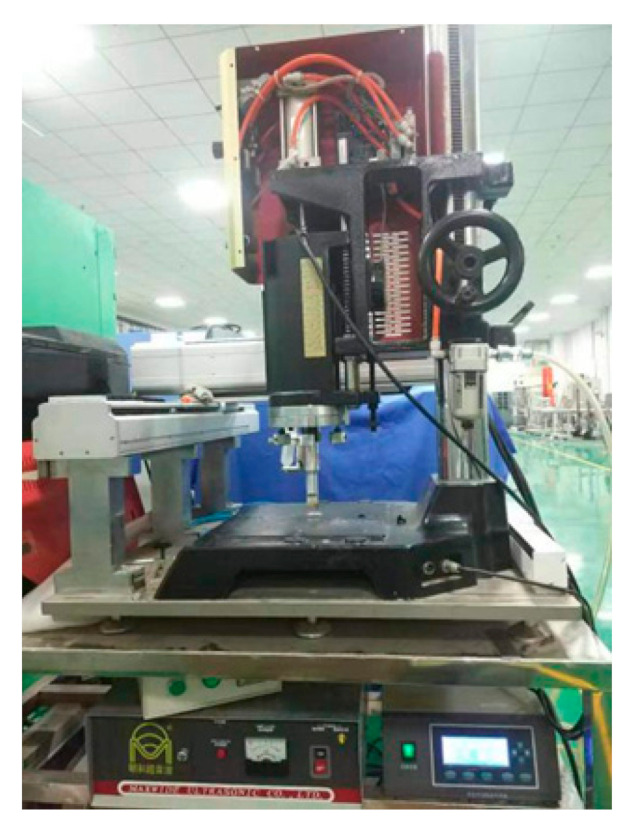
Experiment platform for the ultrasonic treatment.
